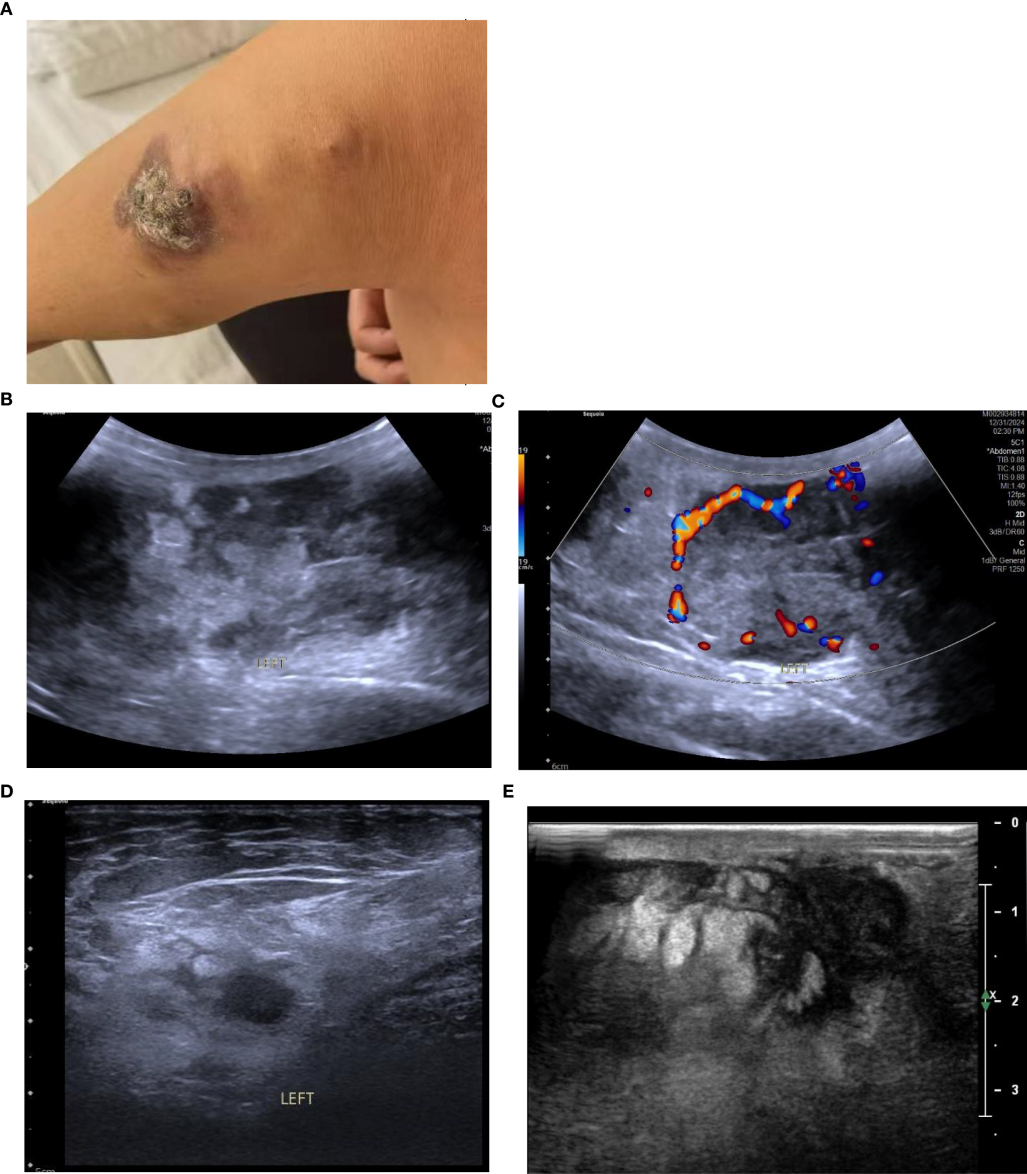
(A) The patient had a protruding red mass on the left upper limb, with a crust on the surface, hard in texture, ill-defined margins, and an irregular shape. (B) Conventional ultrasound revealed multiple hypoechoic solid masses in the subcutaneous tissue of the left upper arm, with ill-defined margins, indistinct edges, and irregular shapes; the largest measures 8.2 × 3.4 cm. (C) CDFI showed relatively rich blood flow signals within the masses. (D) Enlarged lymph nodes were visible in the left axilla, with clear margins and no obvious lymph node structure. (E) After two cycles of chemotherapy, the largest mass measures approximately 4.0 × 2.6 cm (a marked reduction from its pre-treatment size).
